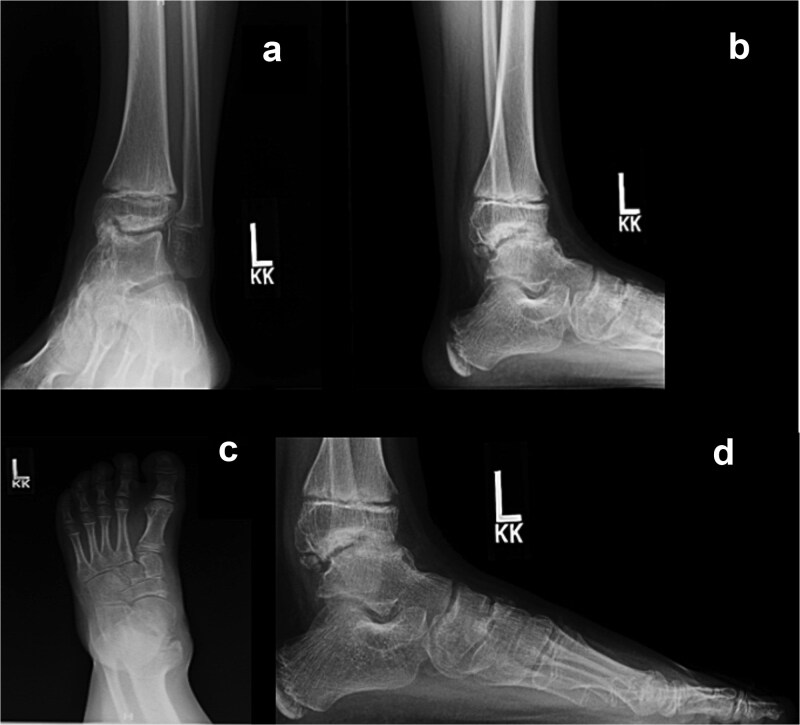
Preoperative AP and lateral ankle (a and b) and foot (c and d) radiographs demonstrating progression of DEH with talar osteochondromas and irregularity of the navicular–medial cuneiform articulation.
